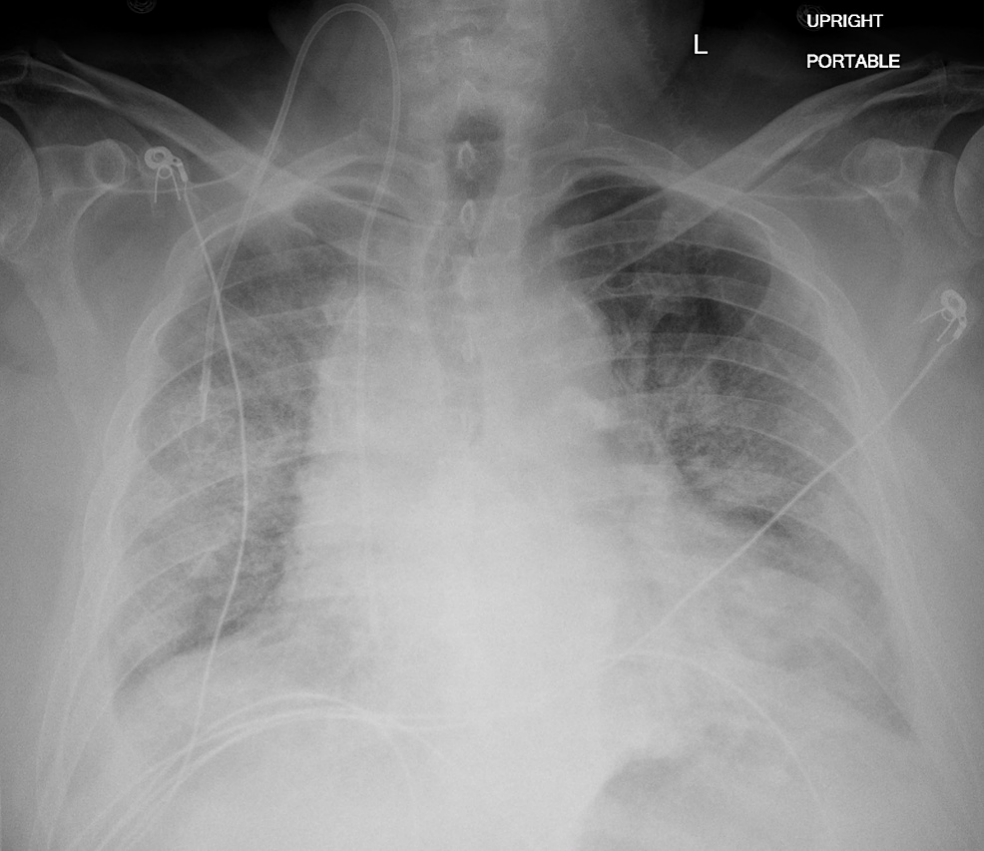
A chest radiograph antero-posterior view - worsening bilateral infiltrates indication acute infectious process.
